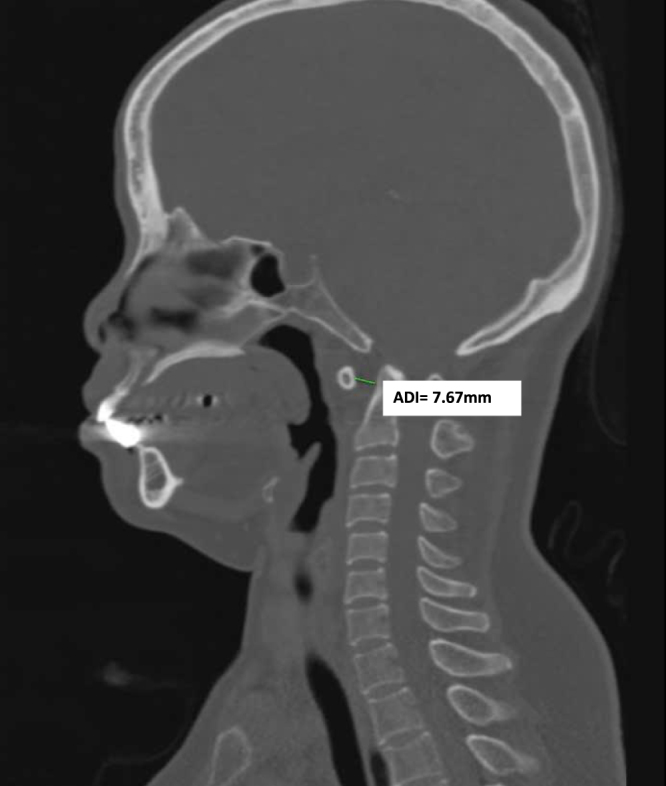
On CT-scan (bone window).
